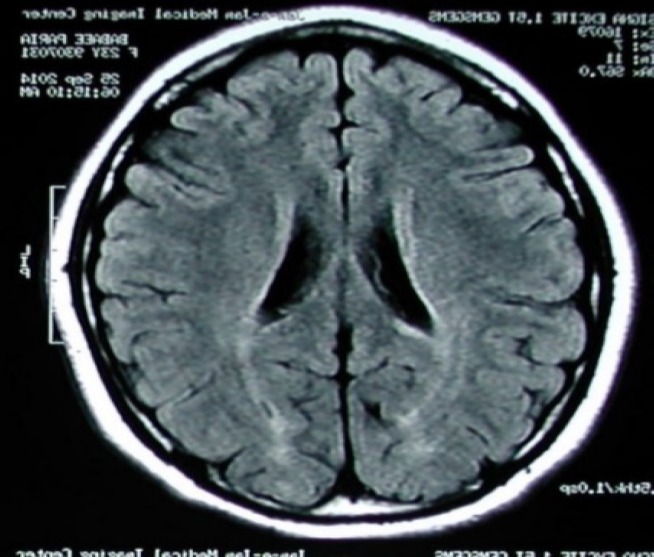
T1 weighted image in magnetic resonance imaging (MRI) of brain (six months follow-up) shows involvement of the basal ganglia and the diencephalon
